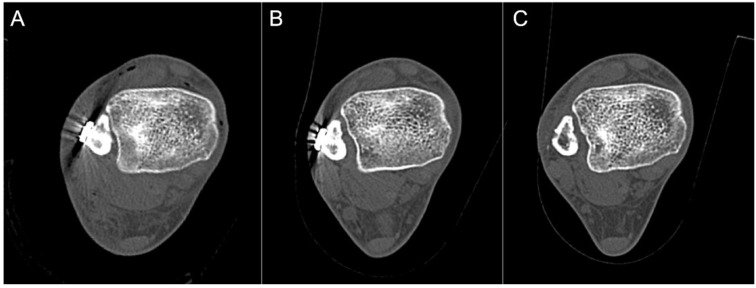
Sequential change of syndesmosis reduction in computed tomography: (A) anteriorly malreduced syndesmosis in immediate postoperative (T1) image. (A/P ratio = 1.5); (B) spontaneous reduction of malreduced syndesmosis a year after initial fixation (T2, just before hardware removal). Note centered fibular in relation to incisura compared to (A) (A/P ratio = 1.2); (C) there was no difference in syndesmosis reduction status three months after suture button removal (T3) (A/P ratio = 1.2). Abbreviations: A/P ratio, anteroposterior measurement ratio.
